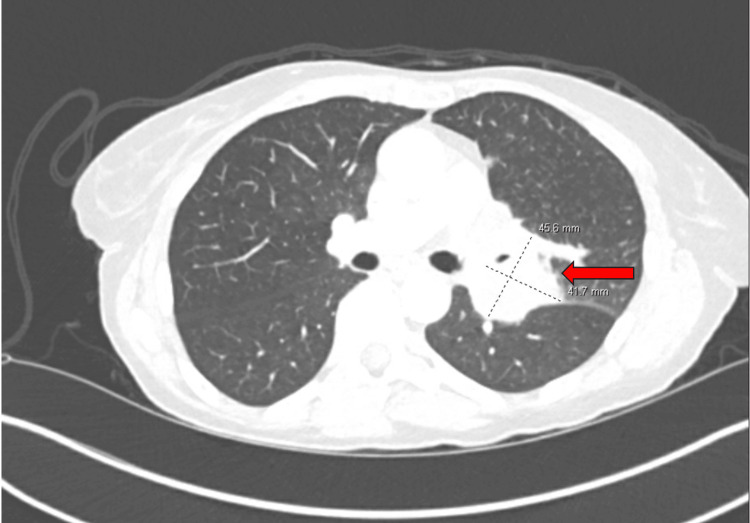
Axial CT of the chest showing left hilar mass and perihilar nodular densities from May 2022. CT: computed tomography
